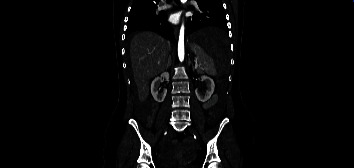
CT abdomen (coronal) demonstrating hemoperitoneum.
